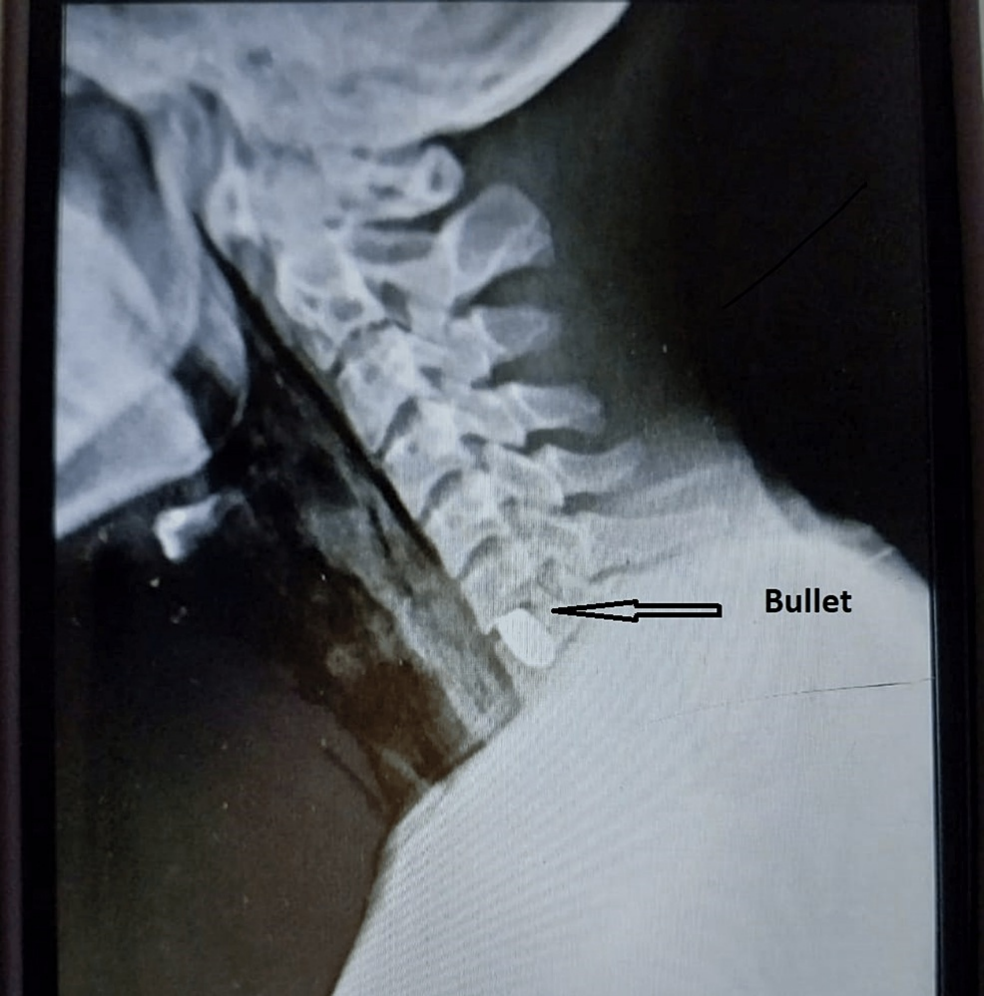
Bullet marked with arrow shows bullet in a lateral x-ray film of the neck.
